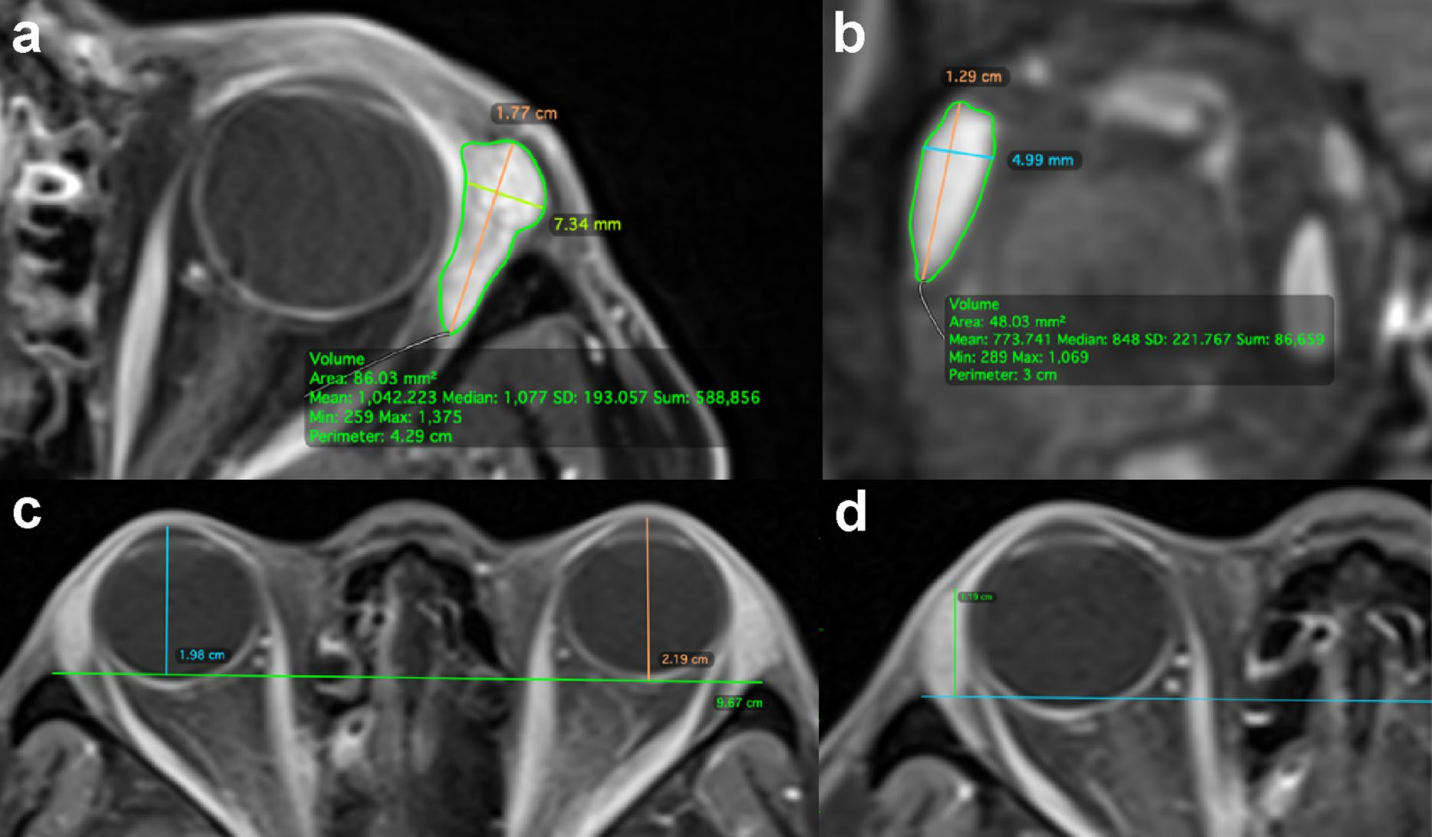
Fat-suppressed contrast-enhanced T1-weighted MRI demonstrating the lacrimal gland quantitative measurements, including, axial length (orange), width (yellow), and area (green) (a), coronal length (orange), width (blue), and area (green) (b), proptosis value (blue/orange) (c), and lacrimal gland prolapse value (green) (d)
